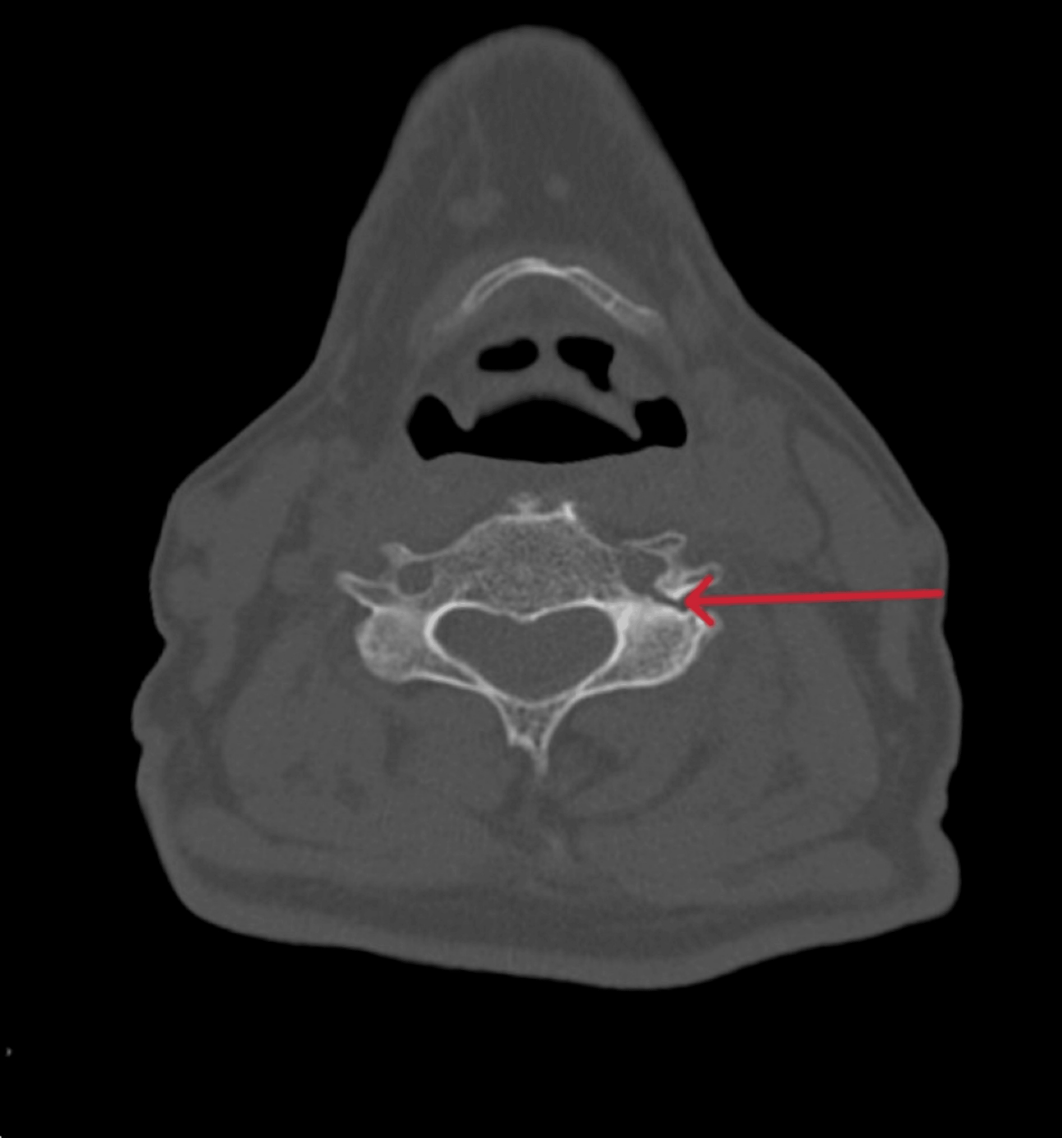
CT scan of the head and cervical spine The red arrow shows a minimally displaced fracture of the left foramen transversarium on an axial view of a non-contrast CT scan of the head and cervical spine.
